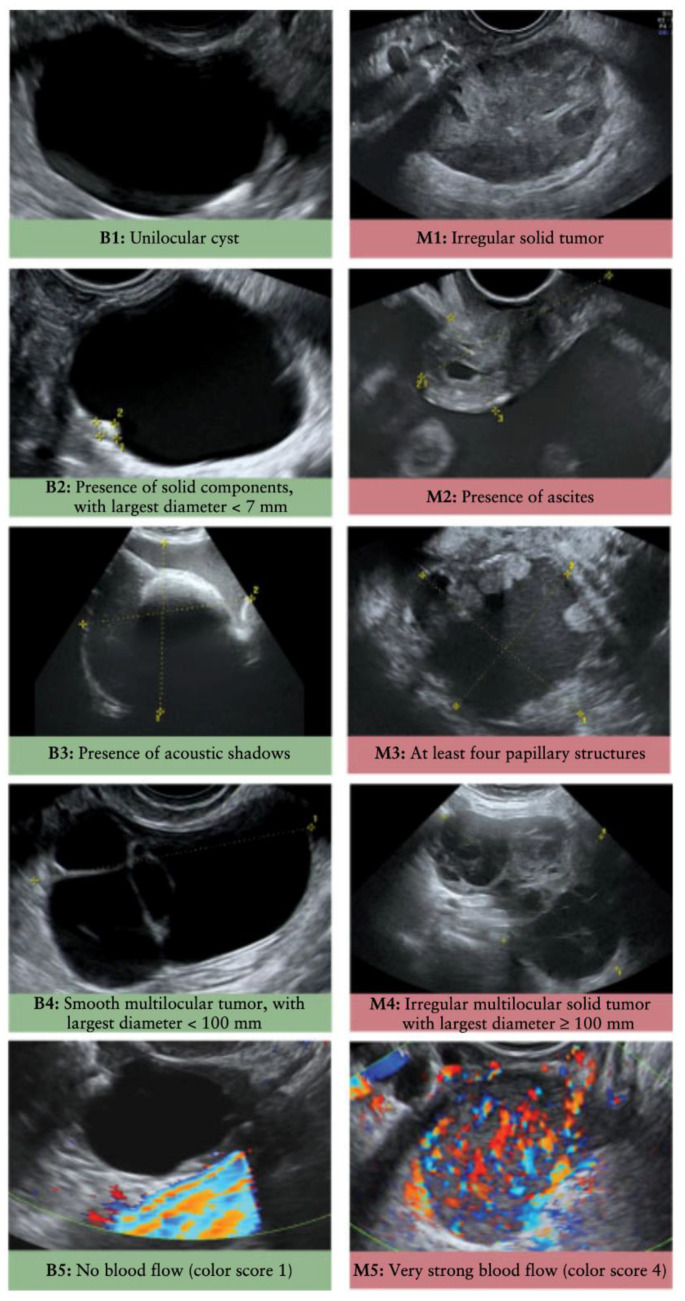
Representative sonographic images of benign (B1–B5) and malignant (M1–M5) ovarian masses. Features in each panel are reviewed based on the IOTA simple rules to evaluate malignancy of ovarian lesions. In general, the presence of irregular shaped bodies, papillary projections, and/or internal blood flow are predictive of malignancy. Images provided from Kaijser et al. [41]. Used with permission.
